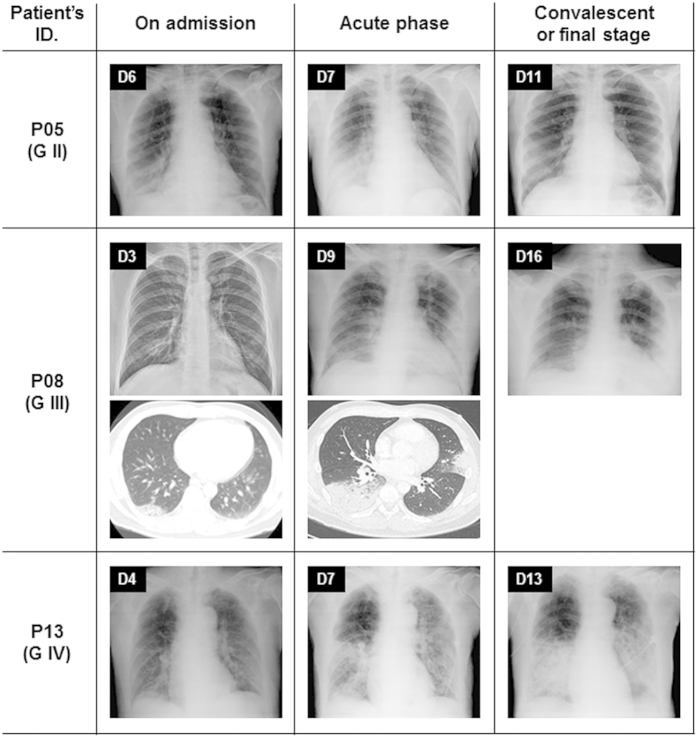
Representative chest images of the MERS patients. Chest images of the MERS patients suffered from mild (P05) or severe pneumonia (P08 and P13) were presented. P05 and P08 were recovered from the pneumonia whereas P13 died due to ARDS. Images were taken at the indicated days after symptom onset. Paired chest radiographs and computed tomography (CT) scans were performed on the same day in P08. Initial chest radiographs shows patchy consolidation along with ground-glass opacities involving unilateral or both lung zones. Follow-up frontal chest radiographs and CT scan (P08) obtained in acute phase show progression in density and extent in all three patients. Chest radiographs taken in convalescent stage show improvement in survived patients (P05 and P08) whereas that of P13 shows progression with bilateral extensive consolidation in both lung zones.
